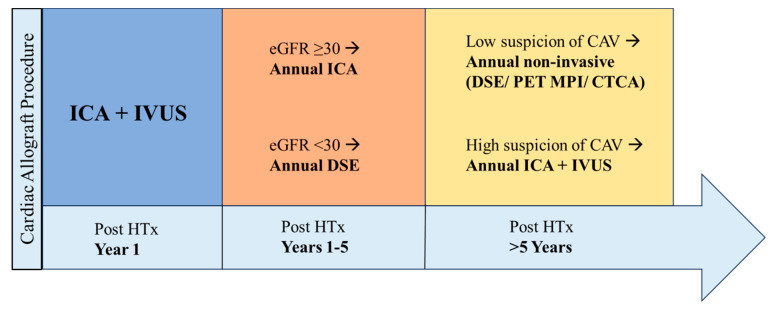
CAV surveillance over time based on expert opinion. *CAV, cardiac allograft vasculopathy; HTx, heart transplant; ICA, invasive coronary angiography; IVUS, intravascular ultrasound; DSE, dobutamine stress echocardiography; PET, positron emission tomography; MPI, myocardial perfusion imaging; CTCA, computed tomography coronary angiography*.
